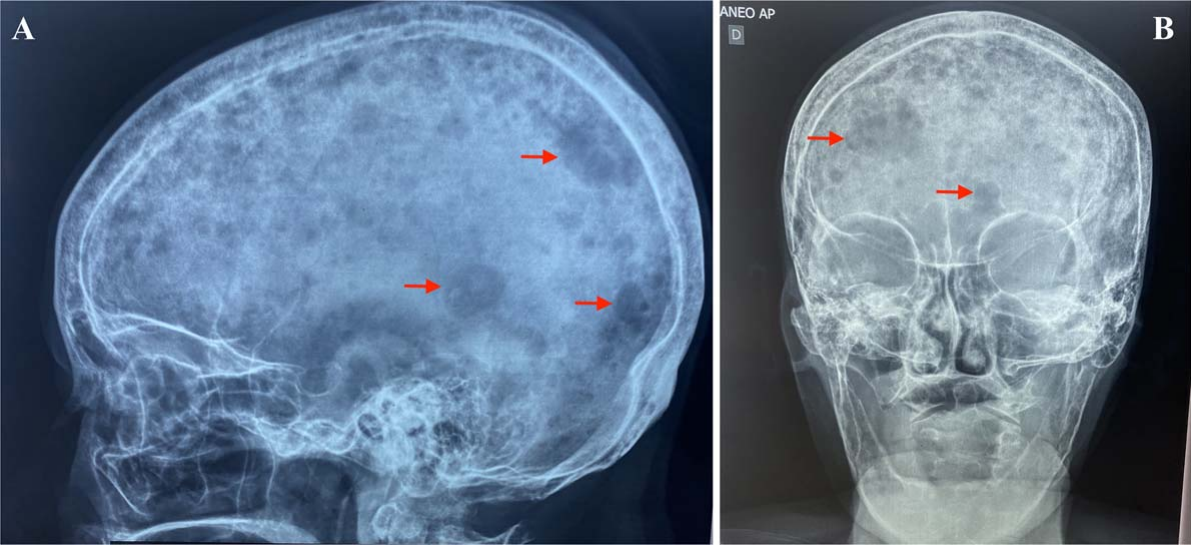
Skull radiographs of a 64-year-old woman with oligosecretory multiple myeloma. (A) Lateral view showing multiple lytic lesions. (B) Anteroposterior view highlighting lytic bone lesions (red arrows).
